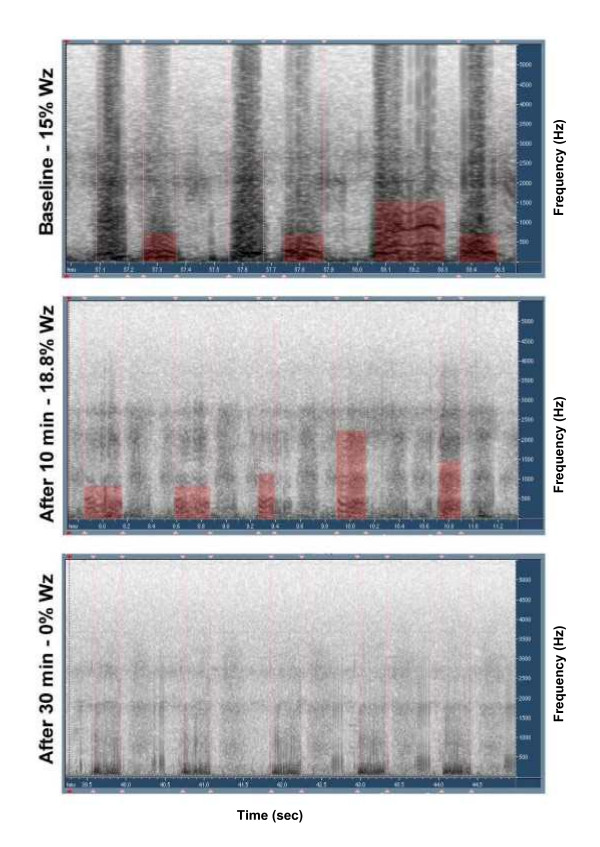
Sonogram of subject treated with epinephrine showing significant reduction in wheezing.
